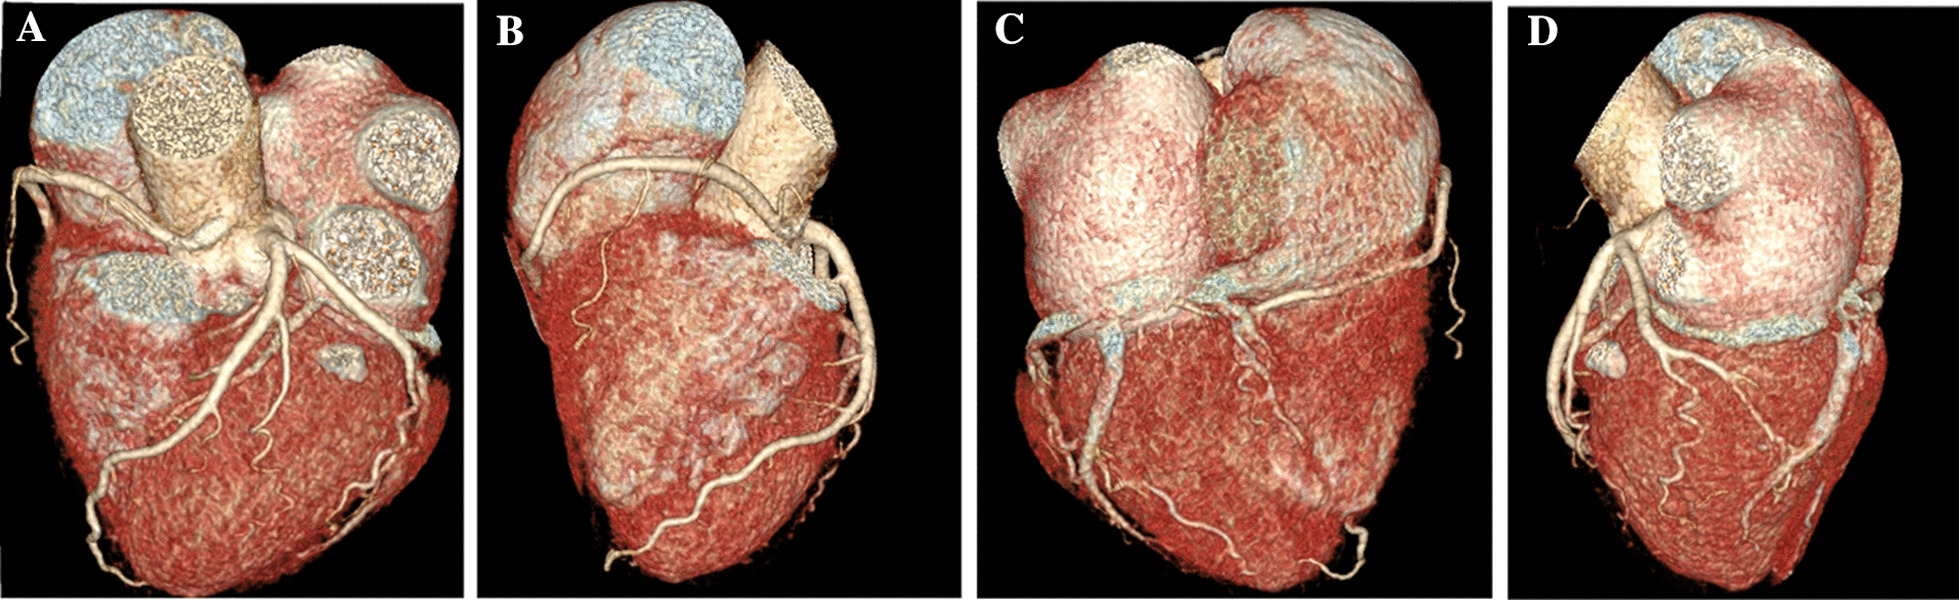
Preoperative  reconstructed 3D computed tomography demonstrating the interarterial course of the right coronary artery. A Anterior view showing the origin left main from the left coronary sinus branching into the LAD and LCx. RCA appears to be entering intramurally into the aortic wall. B Right view of the heart showing the course of the RCA in the atrial-ventricular groove. C Posterior view showing PDA arising from the circumflex. D Left view of the heart showing the LMA, LAD, and LCx. RCA appears to become intramural and no longer visible
